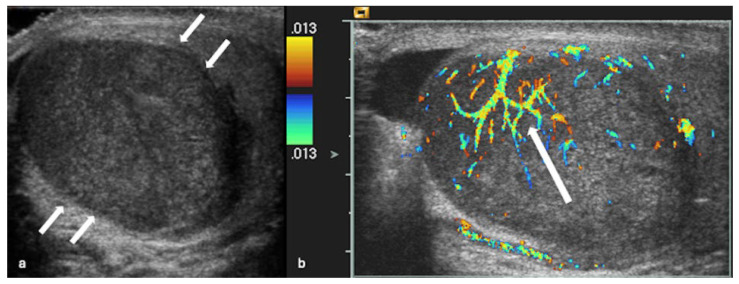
Representative colour Doppler appearances of seminoma. Transverse and longitudinal images of a seminoma in the left testis: (a) grey-scale ultrasound shows a well-circumscribed, hypoechoic focal intratesticular lesion (short arrows); (b) colour Doppler ultrasound demonstrates a criss-cross intralesional vascular pattern (long arrow).
